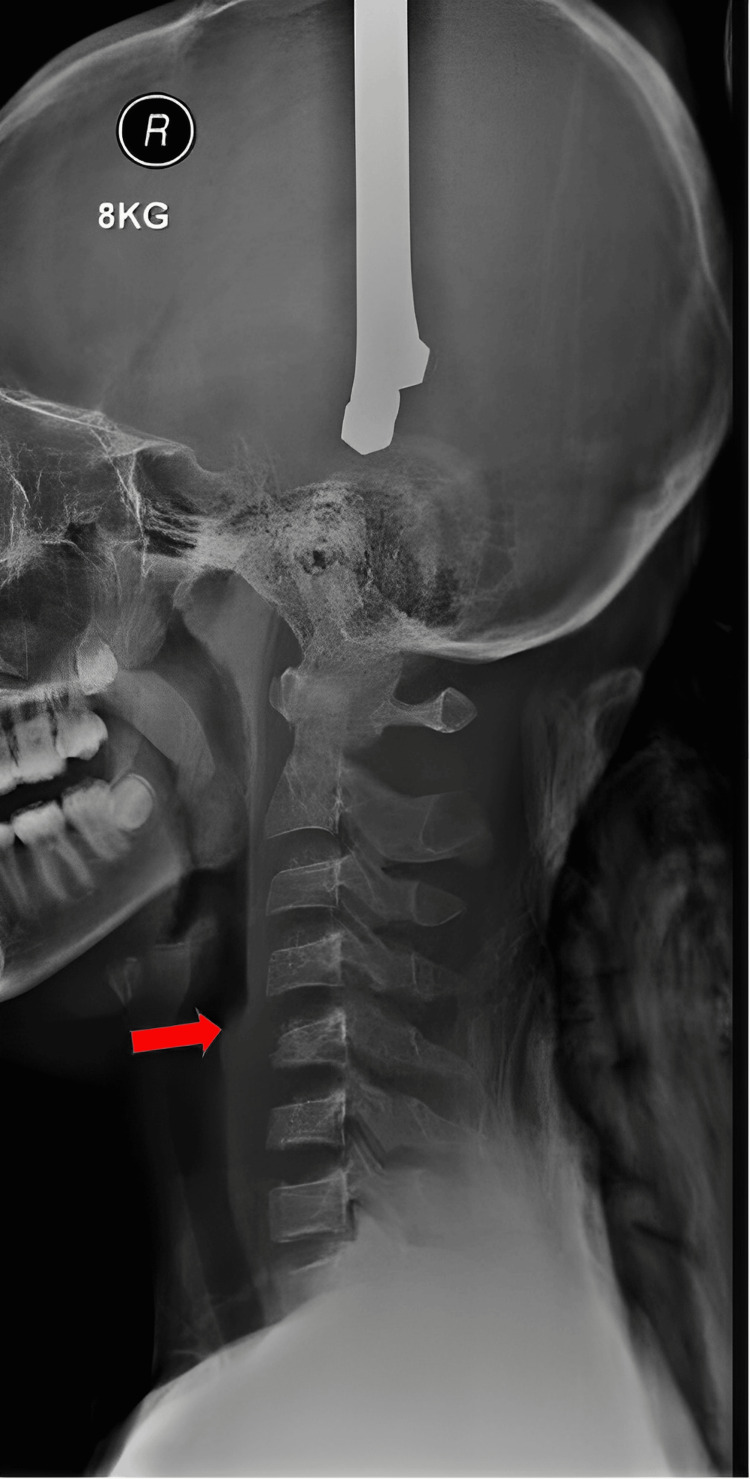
Lateral view of cervical X-ray on 8 kg of traction with red arrow showing reduction of C4/C5 facet dislocation.
